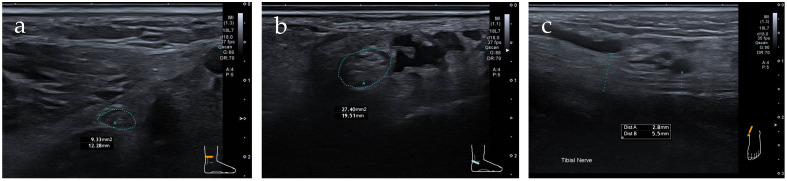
Nerve ultrasound of the tarsal tunnel. (a) Cross-sectional view of the tibial nerve approximately 7 cm proximal to the tarsal tunnel entry (blue circle). (b) Cross-sectional view of the tibial nerve in the proximal portion of the tarsal tunnel (blue circle). Particular attention should be paid to the significant increase in cross-sectional area (CSA) from 9.3 mm^2^ (a) to 27.4 mm^2^ (b). (c) Corresponding longitudinal view of the tibial nerve (right = proximal; left = distal), demonstrating marked caliber enlargement (blue lines) and loss of the fascicular architecture as a correlate of nerve injury.
